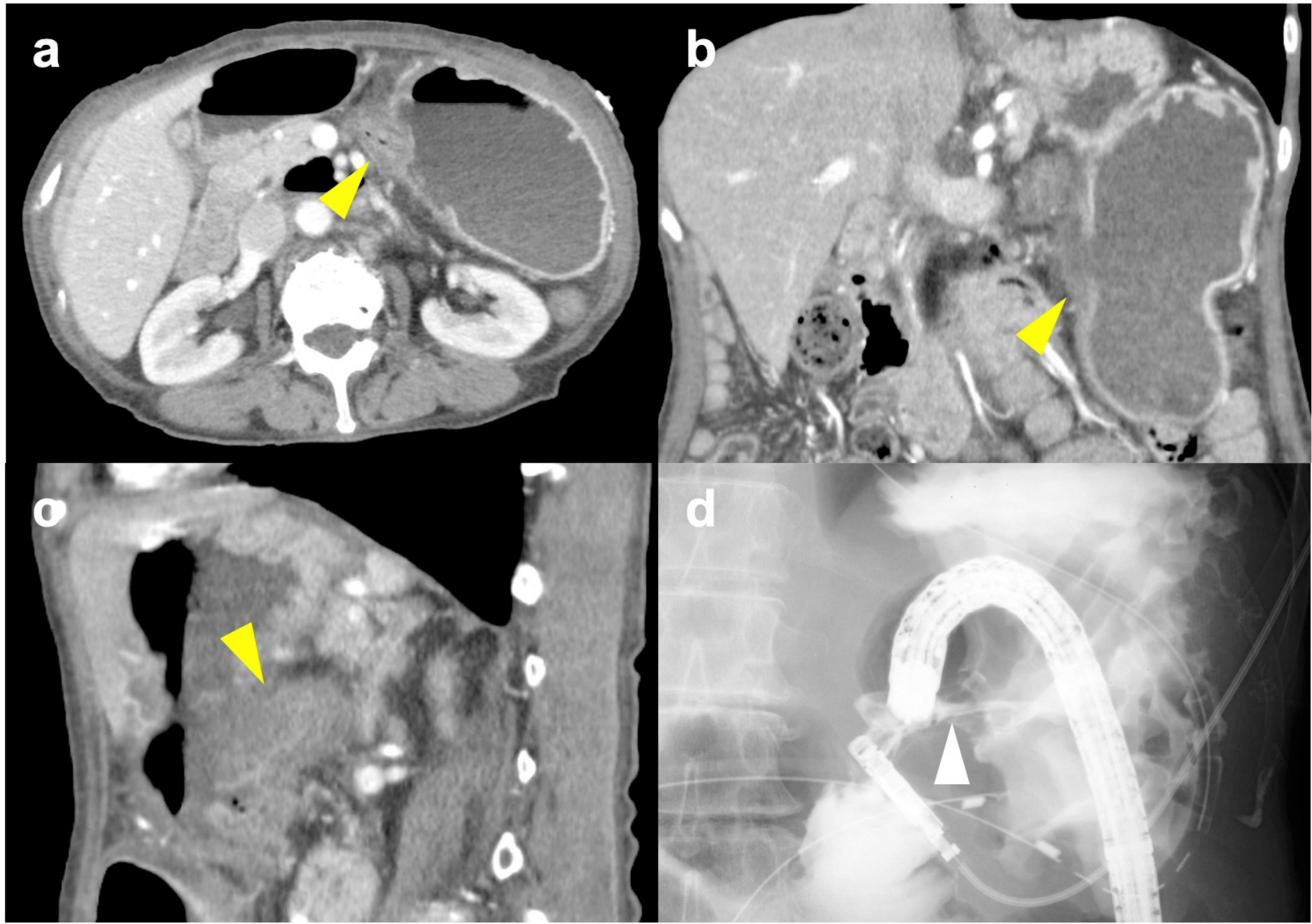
CT scan images and a fluoroscopic image of gastro-colic fistula. (a) Axial, (b) coronal, (c) sagital images from contrast-enhanced abdominal CT scan conducted at the time of admission, showing continuity between the mucosa of the transverse colon and the posterior wall of the mid-body of the stomach was demonstrated (yellow arrowhead). No clear signs of abscess formation were recognized. (d) Fluoroscopic image performed concurrently to lower gastrointestinal endoscopy, utilizing Gastrografin as the contrast agent from the colon, successfully visualizing the stomach through a fistula.
